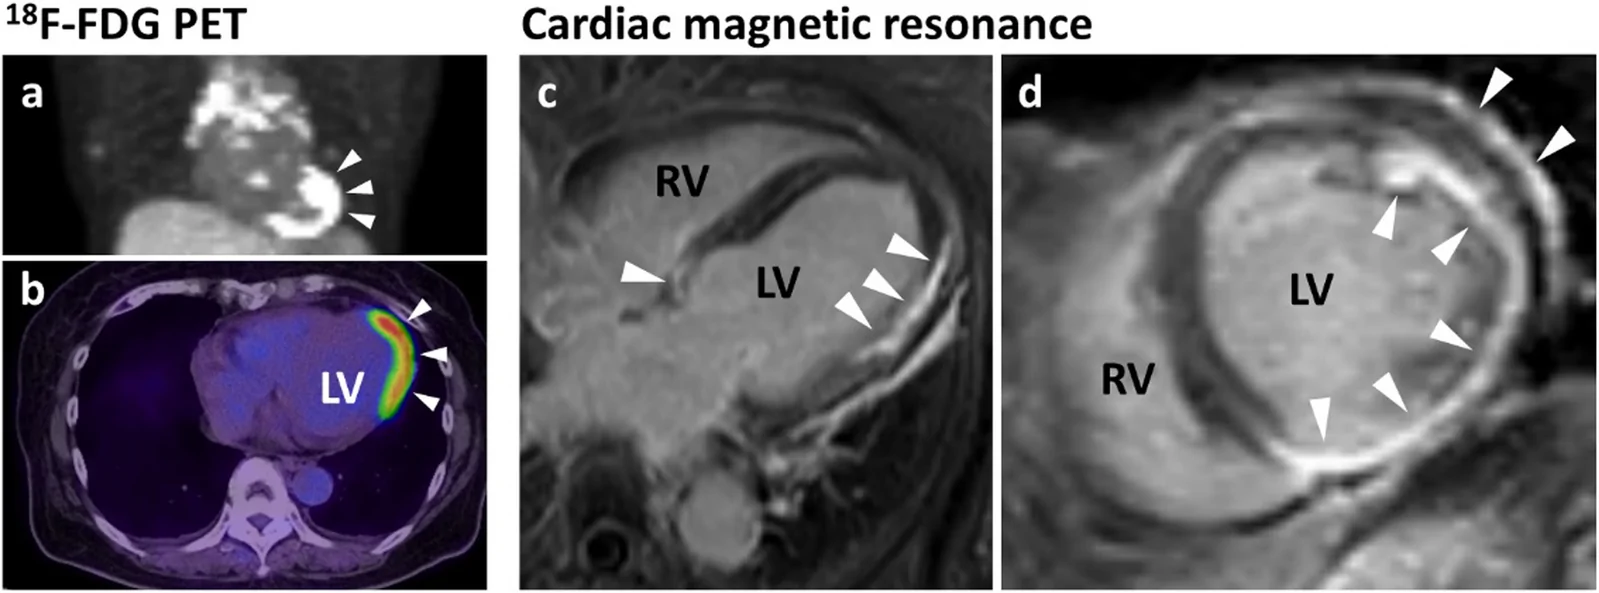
Representative ^18^F-FDG PET and cardiac magnetic resonance images of cardiac sarcoidosis. a, b
